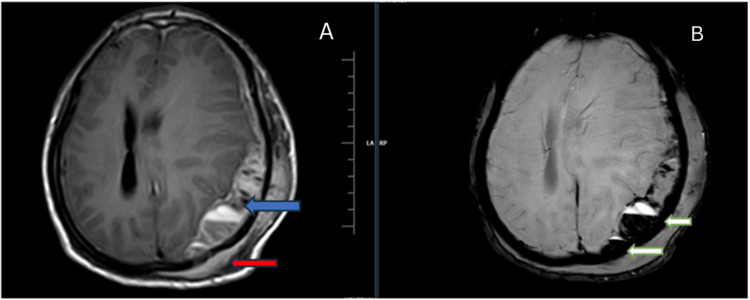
Magnetic resonance imaging brain axial section. (A) T1WI contrast sequence showing heterogeneously enhancing subdural (blue arrow) and extra-calvarial (red arrow) soft tissue lesion in the left frontoparietal region with erosive lysis of the bone; (B) SWI sequence showing fluid levels of blooming (white arrows) suggesting hemorrhage T1WI: T1-weighted image; SWI: susceptibility weighted imaging
